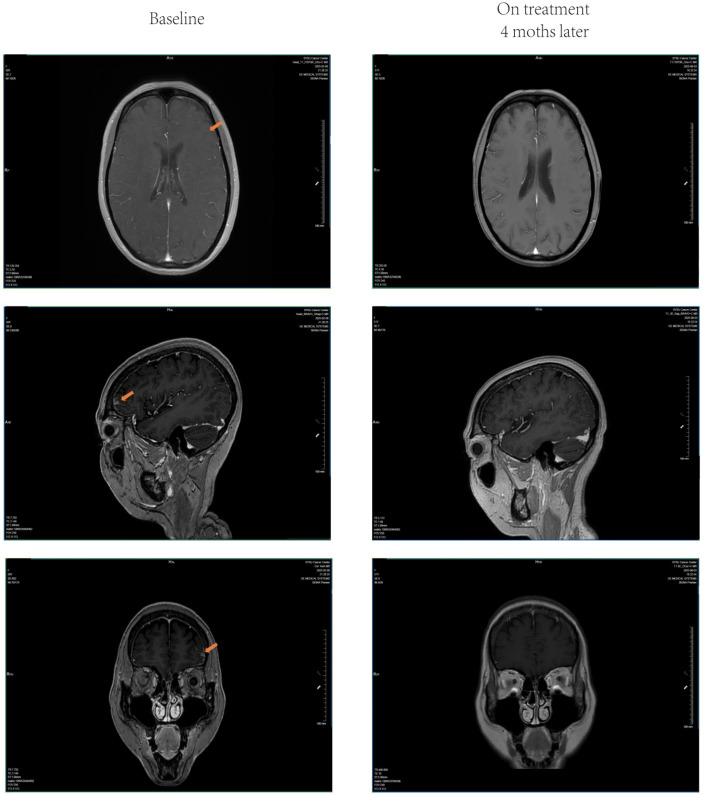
Imaging example of intracranial complete response. A 51-year-old female with melanoma brain metastases treated with a hypofractionated radiotherapy regimen of 6 Gy per fraction for 6 fractions. The magnetic resonance imaging (MRI) shows complete response of the intracranial metastatic lesion at 4-month follow-up. (The orange arrow indicates the lesion).
